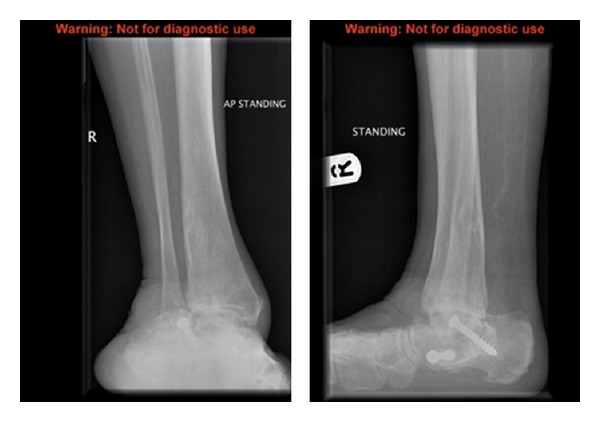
Preoperative radiograph demonstrating previous arthrodesis screws.
